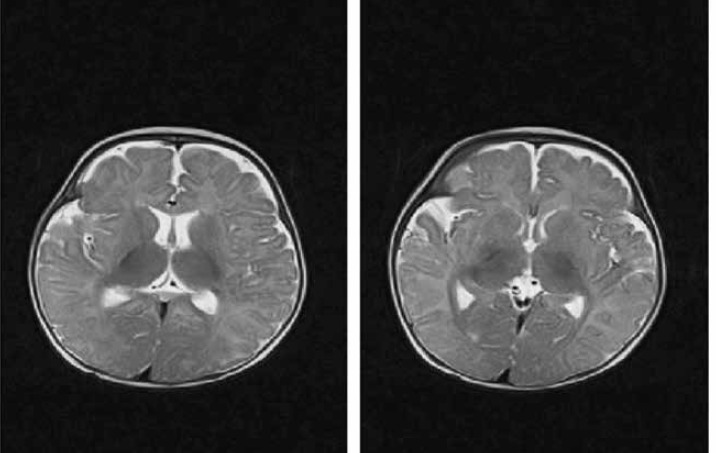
Brain Magnetic Resonance Imaging shows nonspecific low signal intensity in basal ganglia
